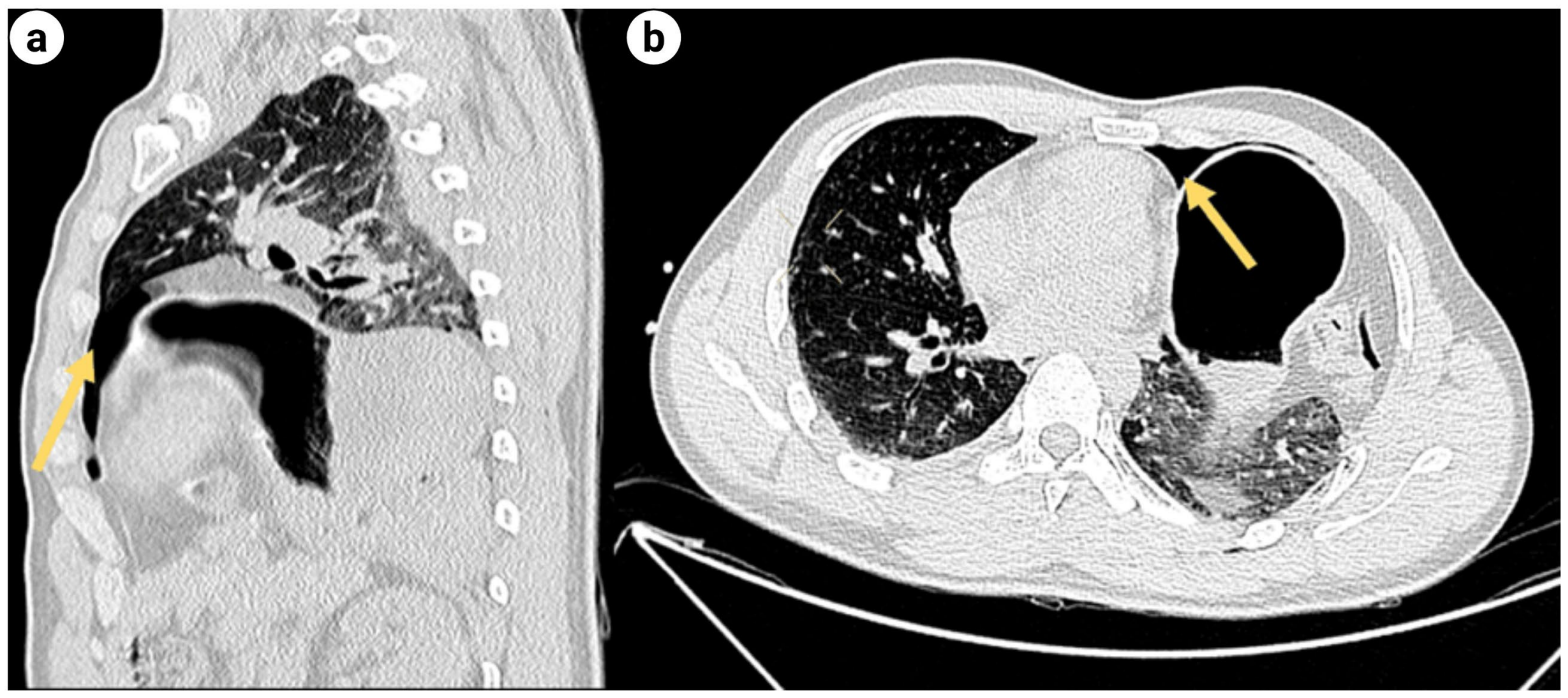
(a) Sagittal and axial CT scans of the chest: Left pneumothorax (denoted by the yellow arrow); (b) the axial CT scan of the chest: Left pleural effusion (indicated by the yellow arrow).
